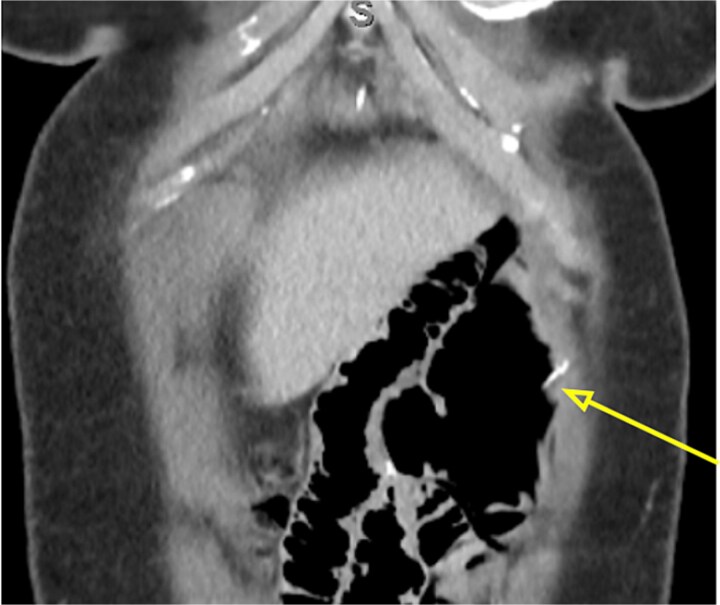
Coronal abdominal CT scan showing the 4 cm hake fishbone (arrow).
